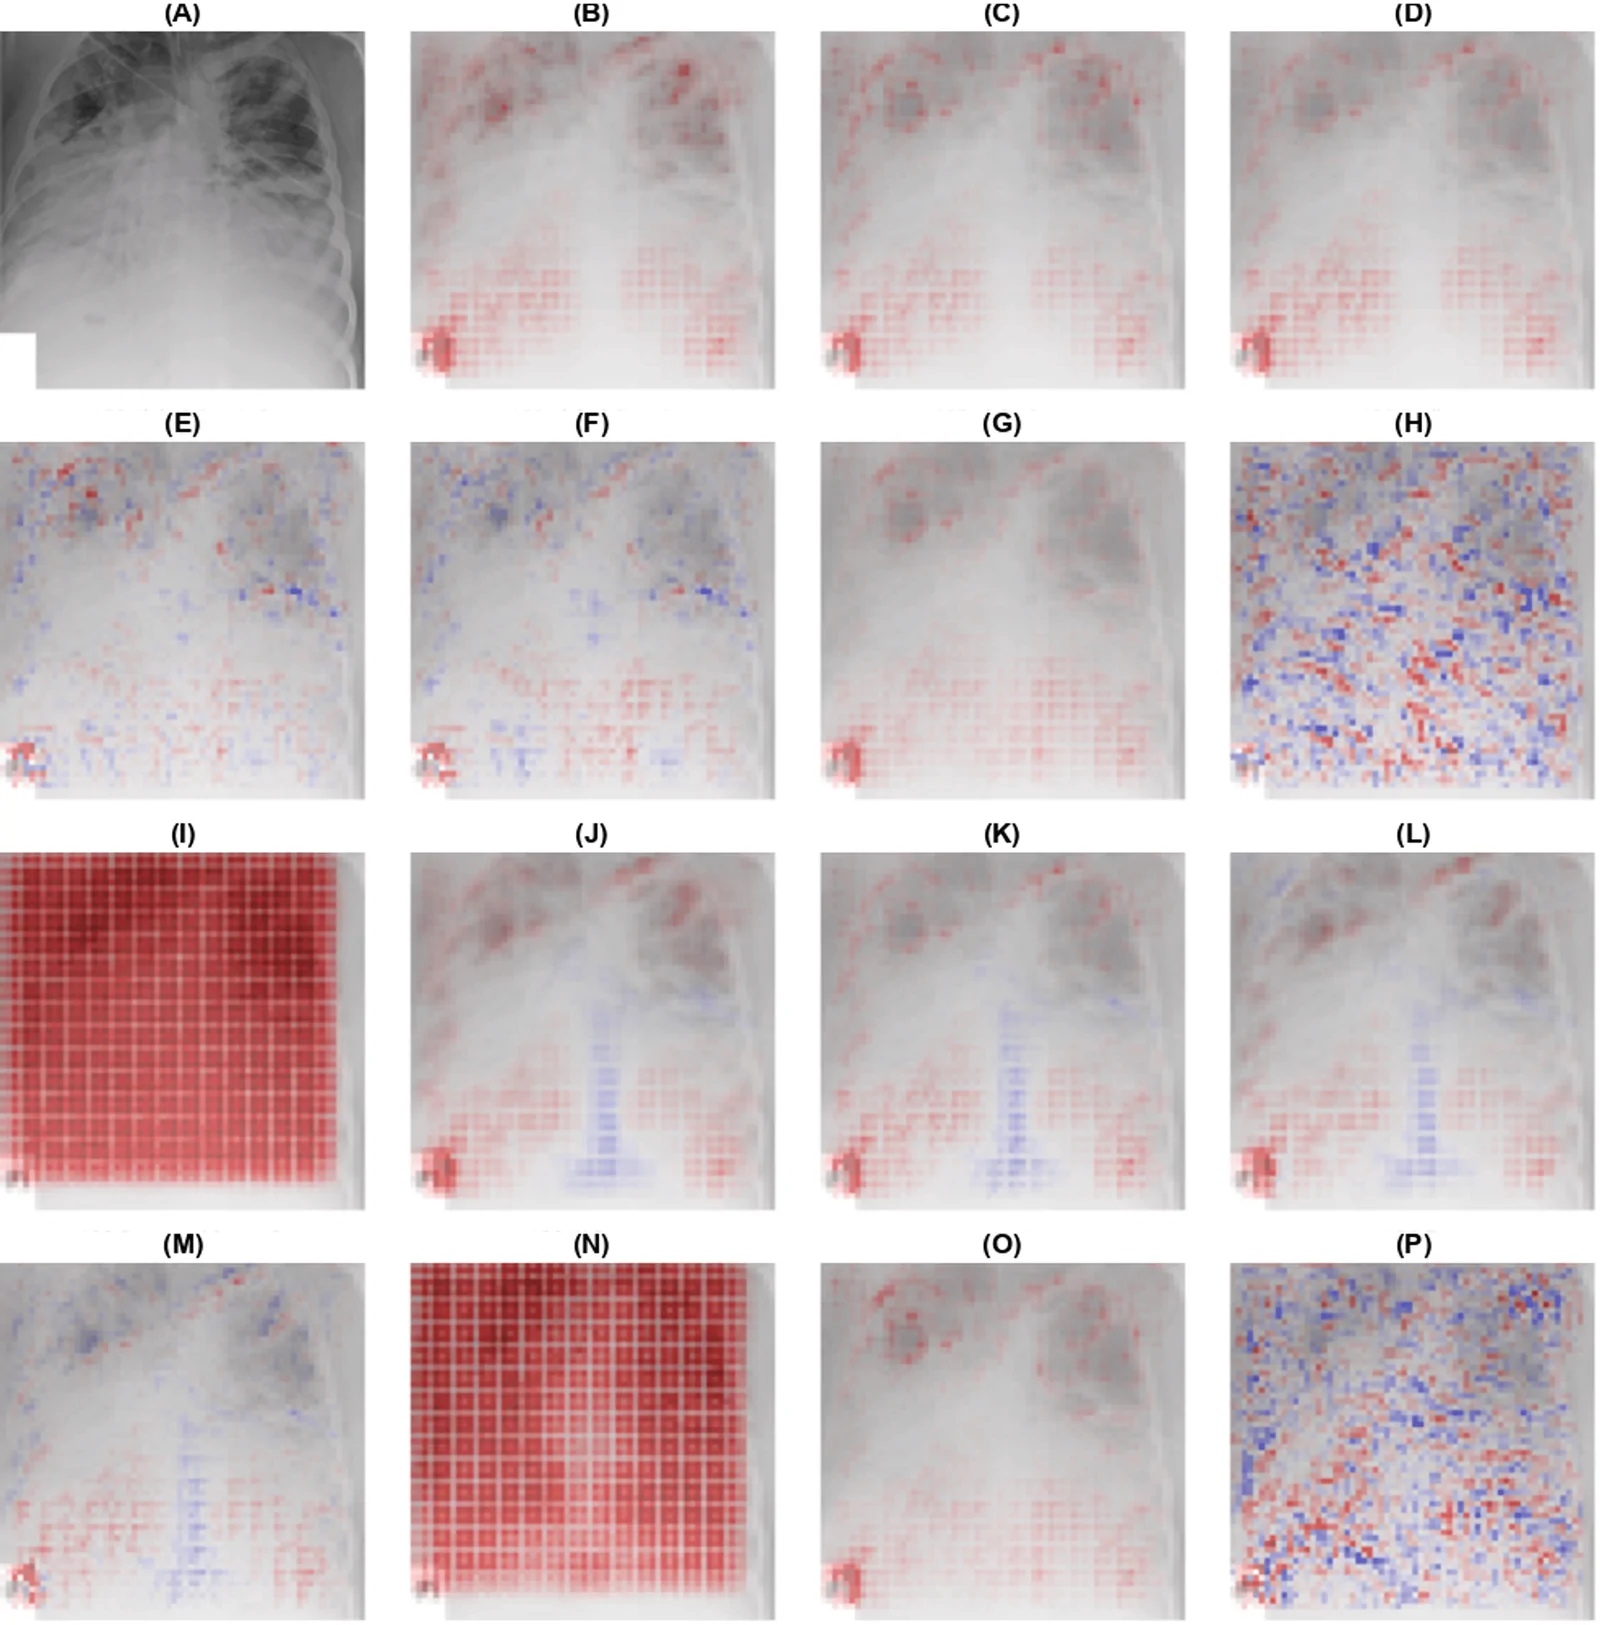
Representative CWOP chest X-ray analyzed with Deep Taylor and LRP rules for the 3-class model. Panels show the original image and corresponding relevance maps generated by each explanation method. (A) Original chest X-ray; (B) Deep Taylor Bounded; (C) Deep Taylor; (D) LRP Alpha 1 Beta 0; (E) LRP Alpha 2 Beta 1 IB; (F) LRP Alpha 2 Beta 1; (G) LRP Alpha Beta; (H) LRP Epsilon; (I) LRP Flat; (J) LRP Sequential Preset A Flat; (K) LRP Sequential Preset A; (L) LRP Sequential Preset B Flat; (M) LRP Sequential Preset B; (N) LRP W Square; (O) LRP Z Plus; (P) LRP Z. COVID-19: coronavirus disease 2019; CWOP: COVID-19 without pneumonia; LRP: layer-wise relevance propagation; IB: input-layer bounded. Panel (A) was adapted from the Covid_w/wo_pneumonia chest Xray dataset [19] and is used under the Community Data License Agreement-Sharing 1.0 (CDLA-Sharing-1.0); all relevance maps and annotations were generated by the authors.
